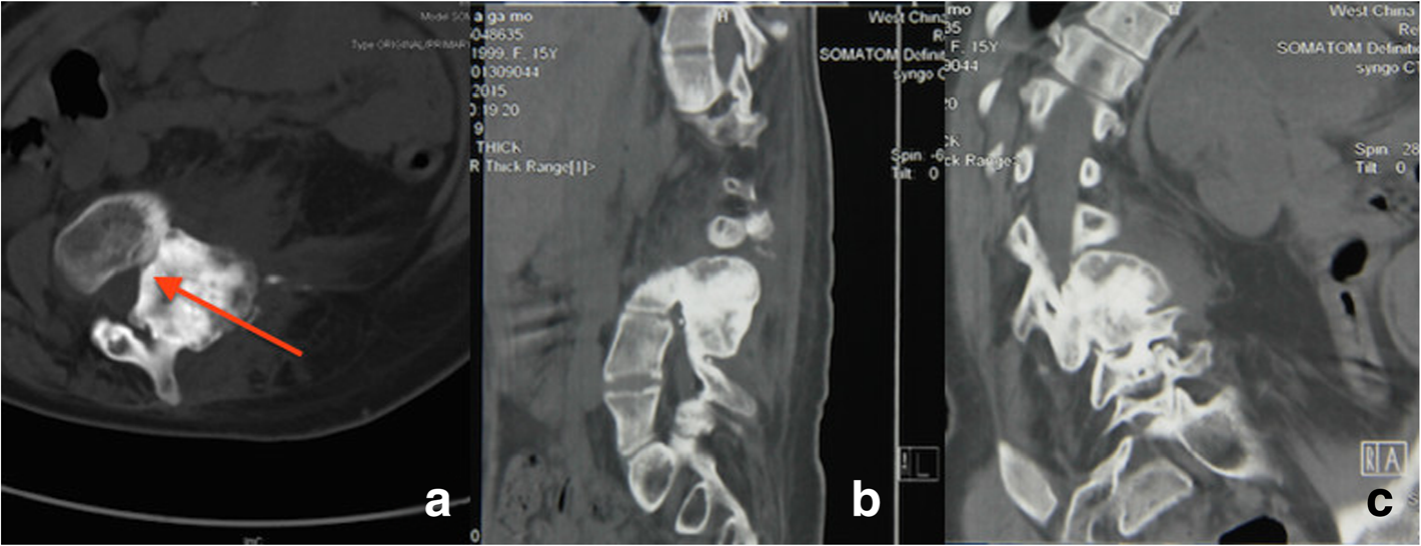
A three-dimensional computed tomography (CT) image of lumbar spine showed a 4*4*5 cm osteoblastic mass located on the left side of L3-L4, presented as a large expansile lesion involving the left pedicle with zygapophyseal joints and partial lamina. The mass had no obvious border to the normal bone tissue. Axial CT showed that the mass occupied the spinal canal and narrowed the nerve root canal of L3/4 (red arrow). a transaxial CT image, b sagittal CT image, c coronal CT image
